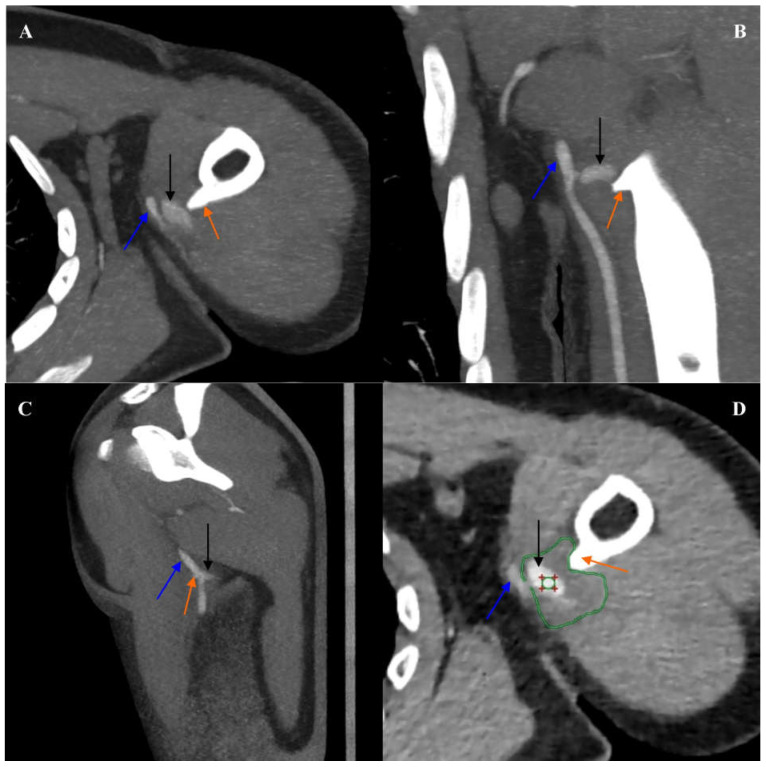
Computed tomography angiography of the left upper limb arteries. Angiographic phase. Multiplanar reconstruction (MPR). (A) Axial view. (B) Coronal view. (C) Sagittal view. (D) Axial view. Soft tissue window. The blue arrow indicates the left brachial artery. The black arrow indicates a pseudoaneurysm of the left brachial artery. The orange arrow indicates the osteochondral exostosis of the left humerus. A green circle with red markers for tissue density measurement was placed within the lumen of the left brachial artery pseudoaneurysm. In the soft tissue window, the borders of the thrombosed part of the pseudoaneurysm of the left brachial artery are indicated with a green outline. A 22-year-old Caucasian man with no significant medical history was admitted to the emergency department due to sudden, intense pain in his left arm, numbness, and pallor of his left forearm and hand. The patient’s consulting vascular surgeon referred him to the computed tomography (CT) laboratory for a computed tomography angiography (CTA) of the arteries of his left upper limb. CTA was performed using a 384-slice Siemens Somatom Force CT scanner (Siemens Healthineers, Erlangen, Germany). The study protocol included a topogram, premonitoring, monitoring at the level of the aortic arch with measurement of contrast enhancement of the region of interest (ROI), an angiographic phase triggered when the ROI density of 100 HU was reached, and a delayed phase 60 s after contrast agent administration. The patient was administered 100 ml of iodinated, non-ionic contrast agent (Iomeron 400, Bracco UK Ltd., Oxford, UK) via an automated syringe into the right antecubital vein at an infusion rate of 4.0 mL/s. Axial reconstructions were obtained with slice thicknesses of 5.0 and 0.6 mm. Secondary multiplanar reconstruction (MPR) and volume rendering technique (VRT) reconstruction were performed. In the CTA examination, at the level of the proximal segment of the left brachial artery, lateral to the brachial artery, above the proximal segment of the branching left deep brachial artery, an excess of contrast was visualized, measuring up to approximately 1.5 × 1.2 cm in cross-sections and up to approximately 0.7 cm in the craniocaudal dimension, connecting with the left brachial artery by narrow bands of approximately 0.1 cm and 0.3 cm in width. The CTA image was suggestive of a pseudoaneurysm of the left brachial artery. In the soft tissue window of the CTA, around the above-mentioned excess contrast, a thrombosed component of the pseudoaneurysm was differentiated within the muscles of the left arm, with approximate overall dimensions of 3.4 × 3.2 cm in cross-sections and 2.9 cm in the craniocaudal dimension.
